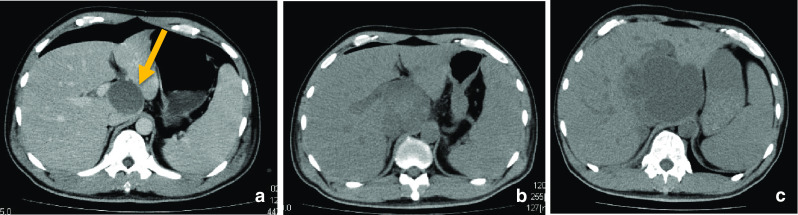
Abdominal CT revealed new cystic lesions appeared in liver segment S1 (a, arrow) and S7 (not shown) 4 days after surgery. These lesions grew rapidly 11 days after surgery (b). The fine needle aspiration cytology revealed that these were metastatic lesions from the undifferentiated carcinoma with rhabdoid features. Chemotherapy was ineffective and they continued to progress 47 days after surgery (c)
